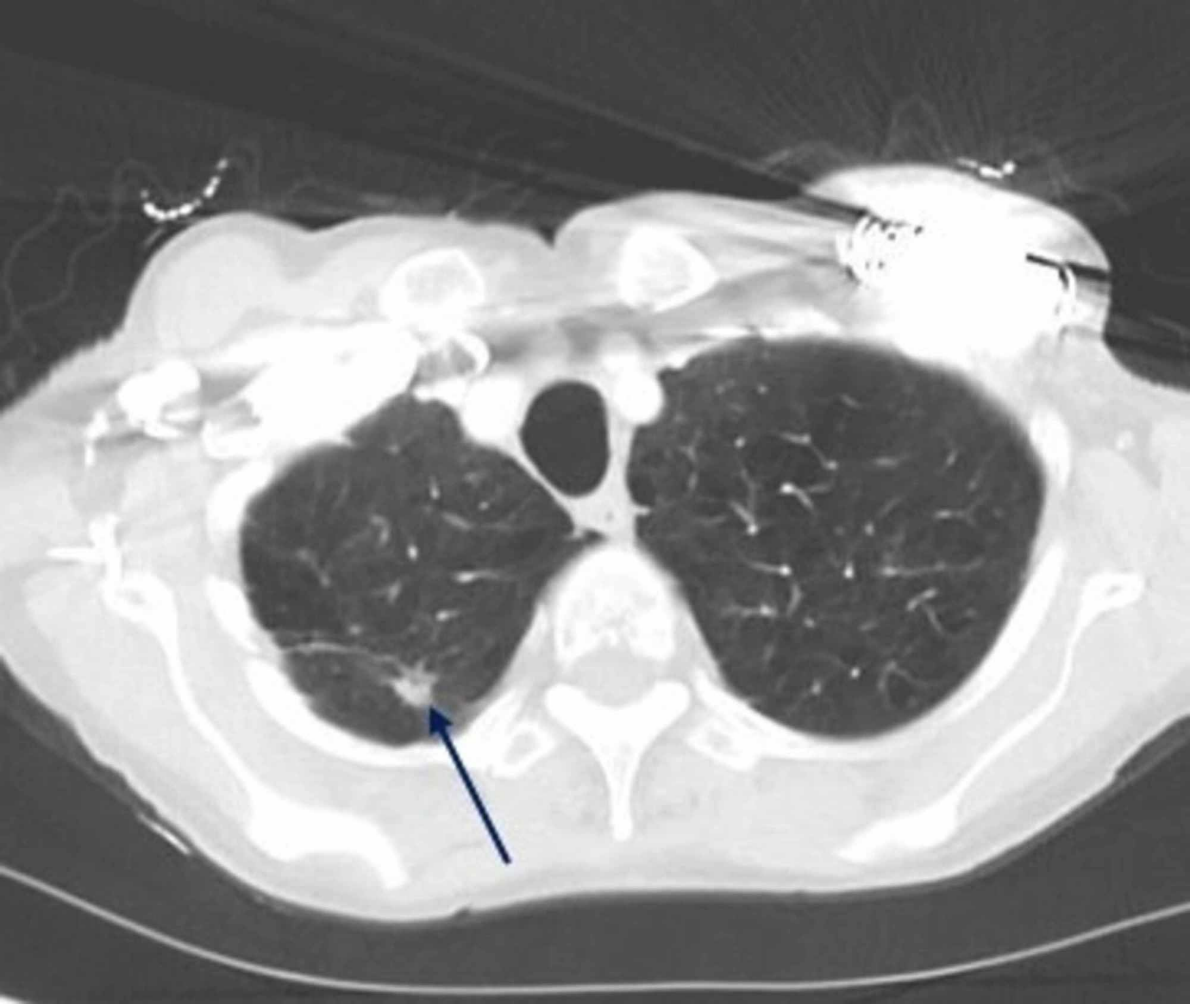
CT-thorax showing a right upper lobe malignant lung nodule (blue arrow), after radiation therapy
